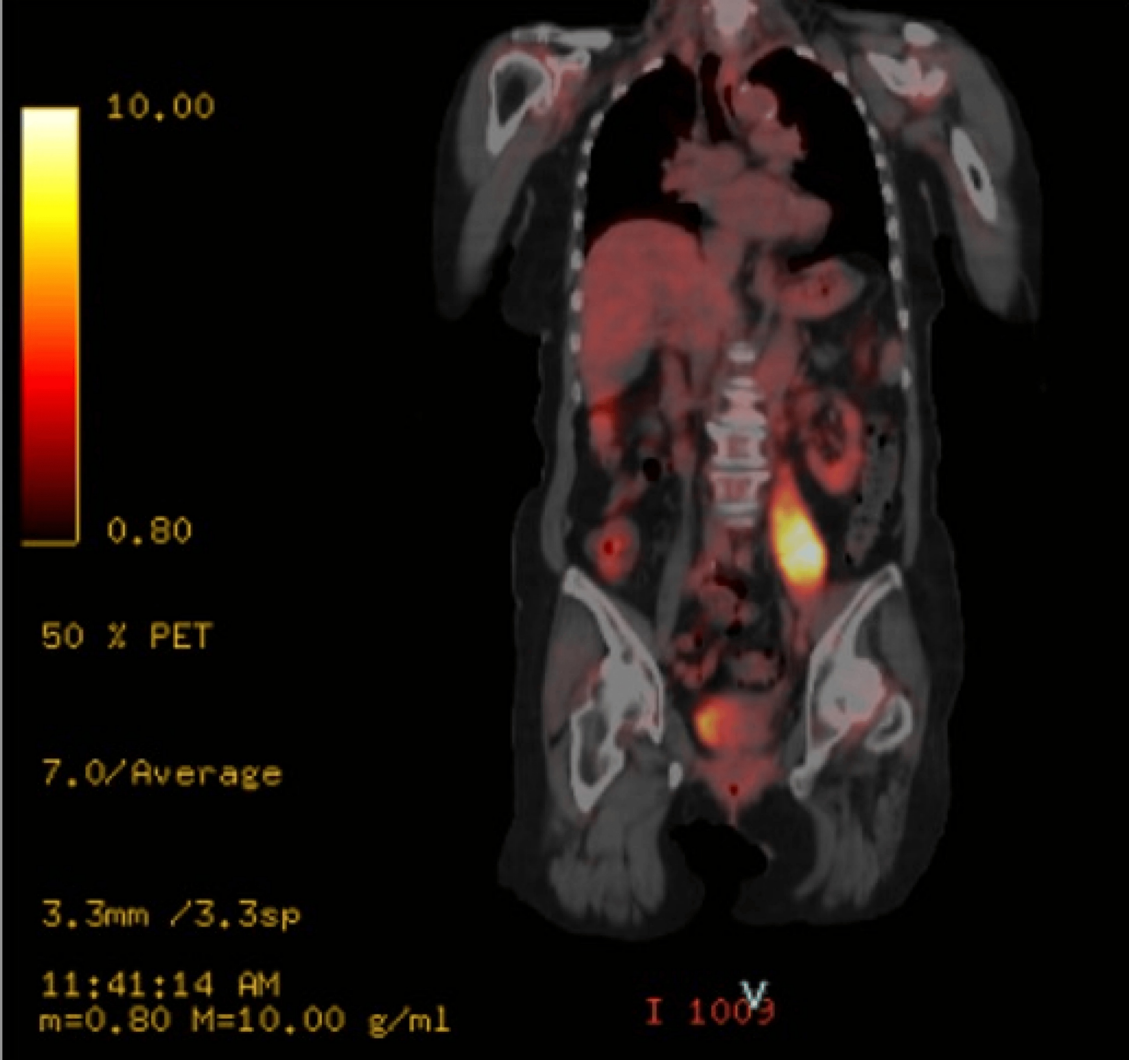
Positron emission tomography-computed tomography findings. Abnormal accumulation in the left psoas muscle (maximum standardized uptake value = 11.2), suggestive of malignancy rather than an abscess.
